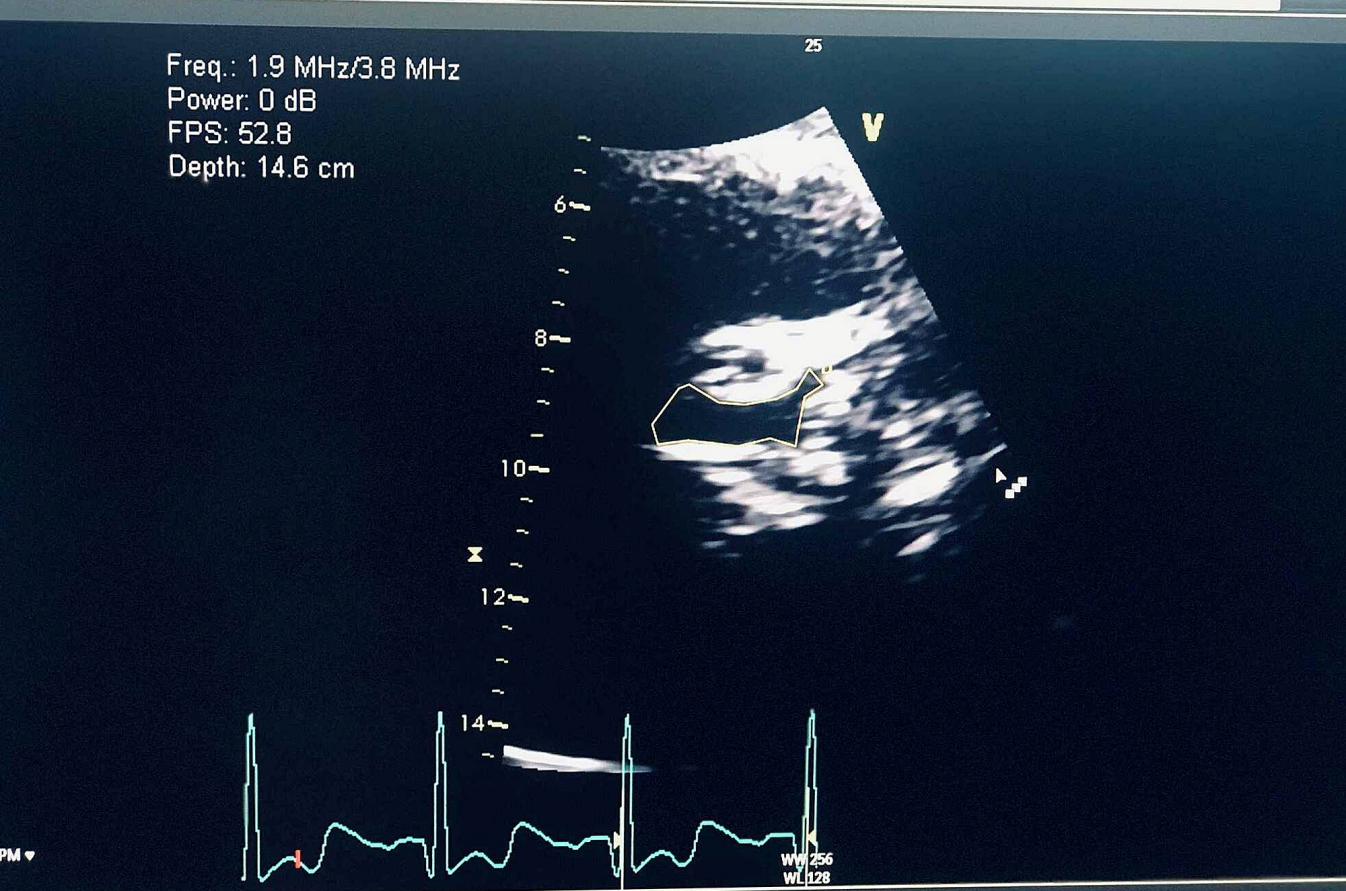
Transthoracic echocardiogram showing moderate calcification of the aortic leaflets Area demarcated by yellow line shows aortic stenosis.
